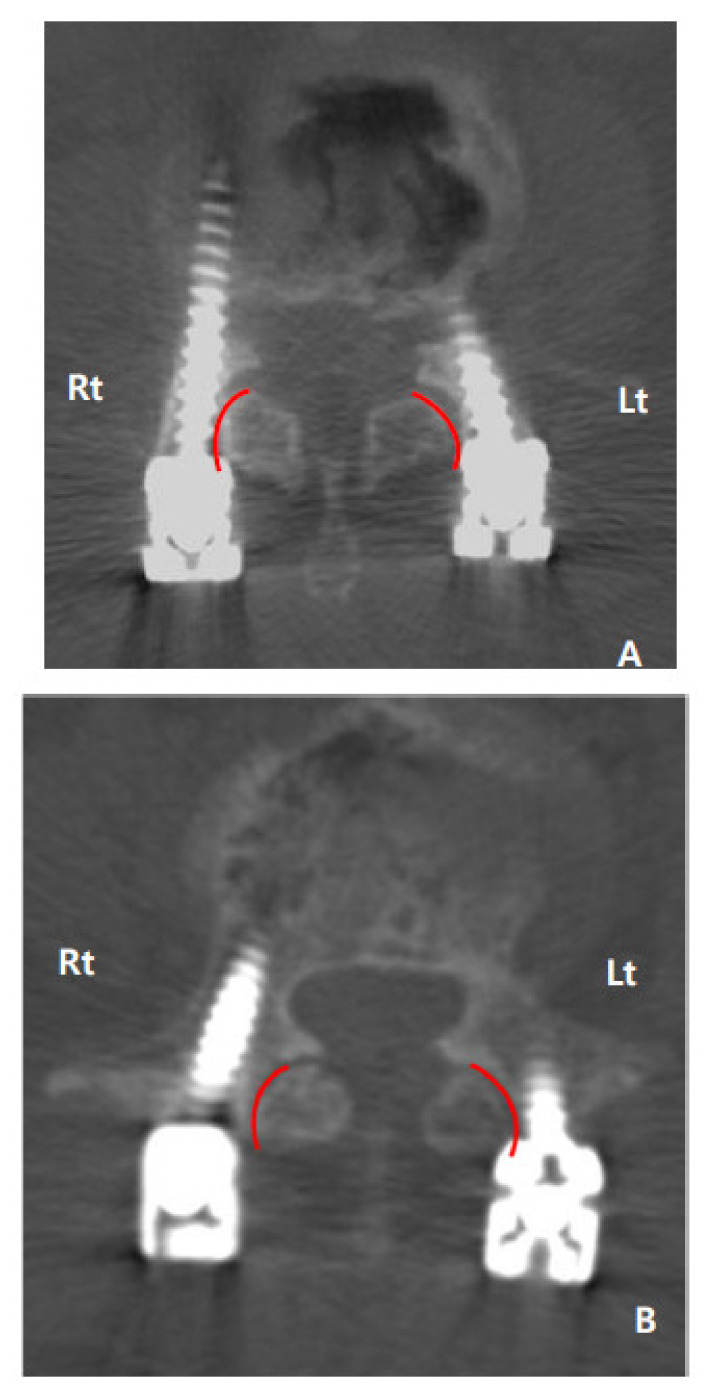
Superior facet joint violation by transpedicular screw evaluated using computed tomography. (A) The right is 2 points, the left is 1 point, and the final score is 3 points; (B) the right is 0 point, the left is 2 points, and the final score is 2 points.
